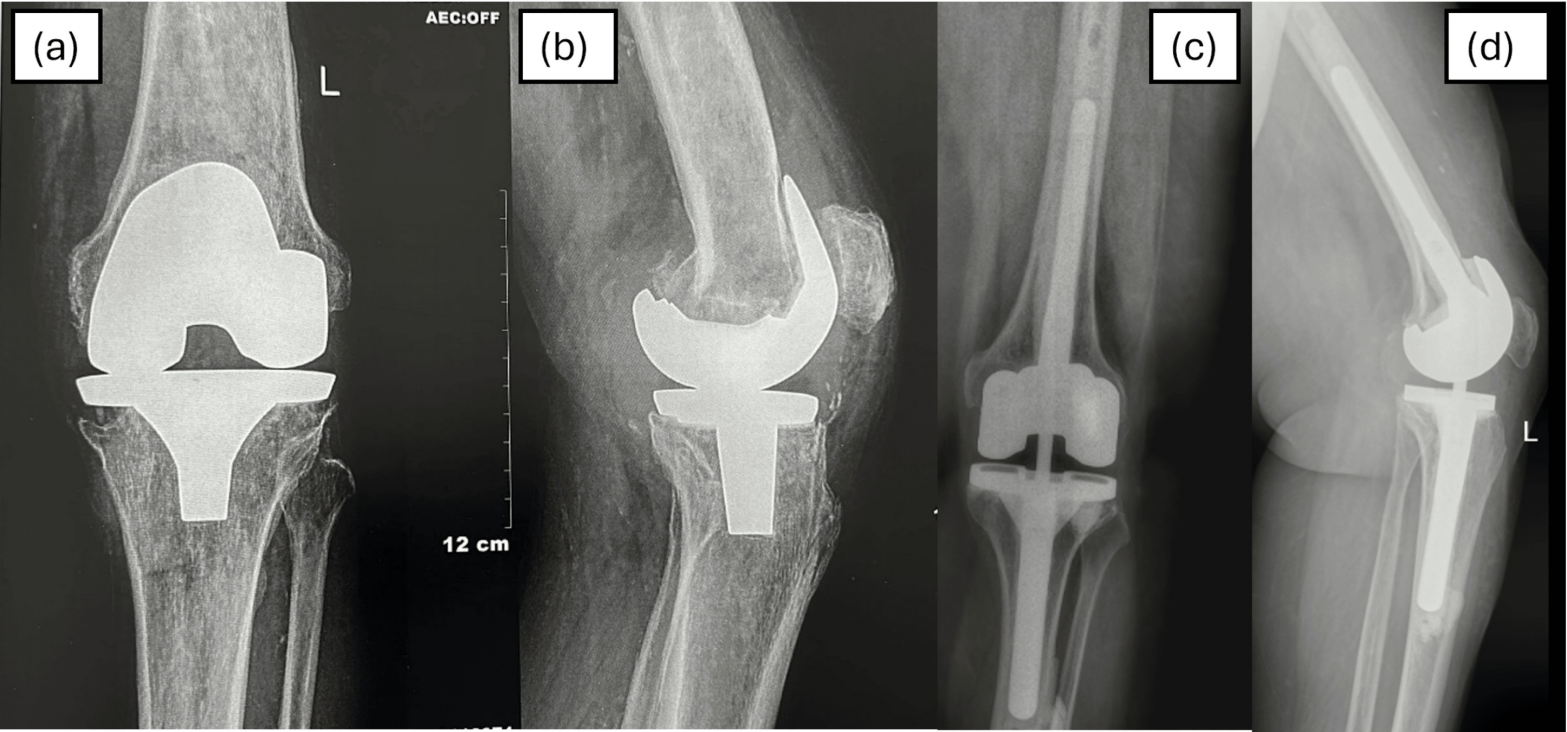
Pre-operative AP (a) and lateral (b) radiographs of the left knee showing aseptic loosening of the femoral and tibial components; AP (c) and lateral (d) radiographs of the same knee after a single-stage revision surgery using the TC-3 prosthesis, showing well-fixed femoral and tibial stems at the final six-year follow-up
